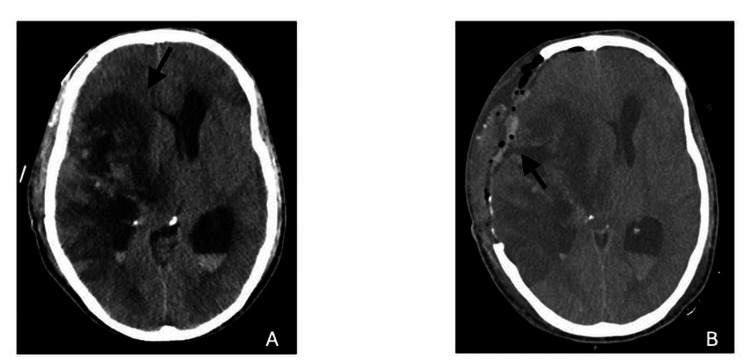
(A) Brain CT scan showing right frontal and parietal cortico-subcortical hypodensities and global increase in right frontal-temporo-parietal lesional edema. (B) Brain CT scan after decompressive craniectomy.
